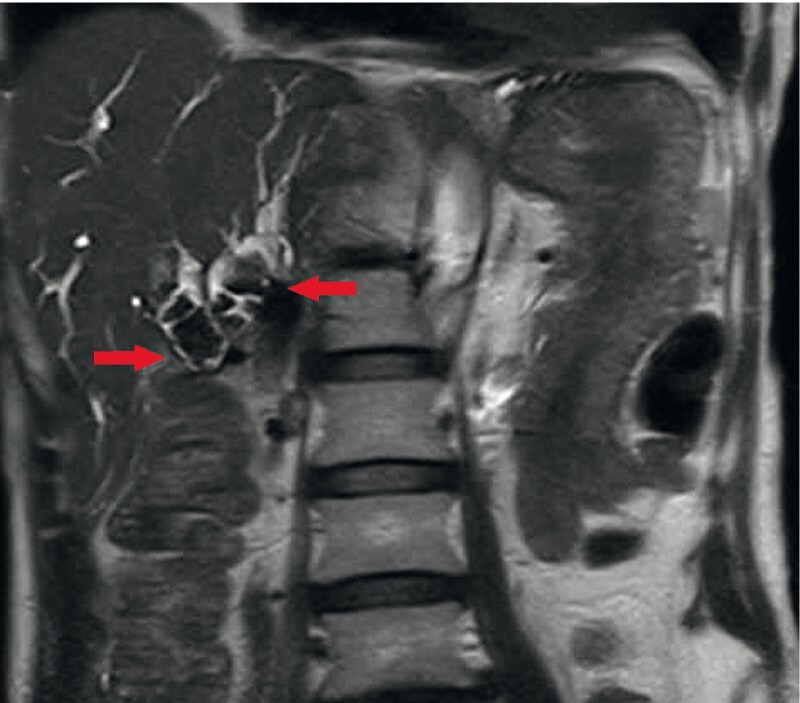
Magnetic resonance imaging revealed dilatation and multiple large stones in the bilateral intrahepatic bile duct. The red arrow shows multiple large bile duct stones.
